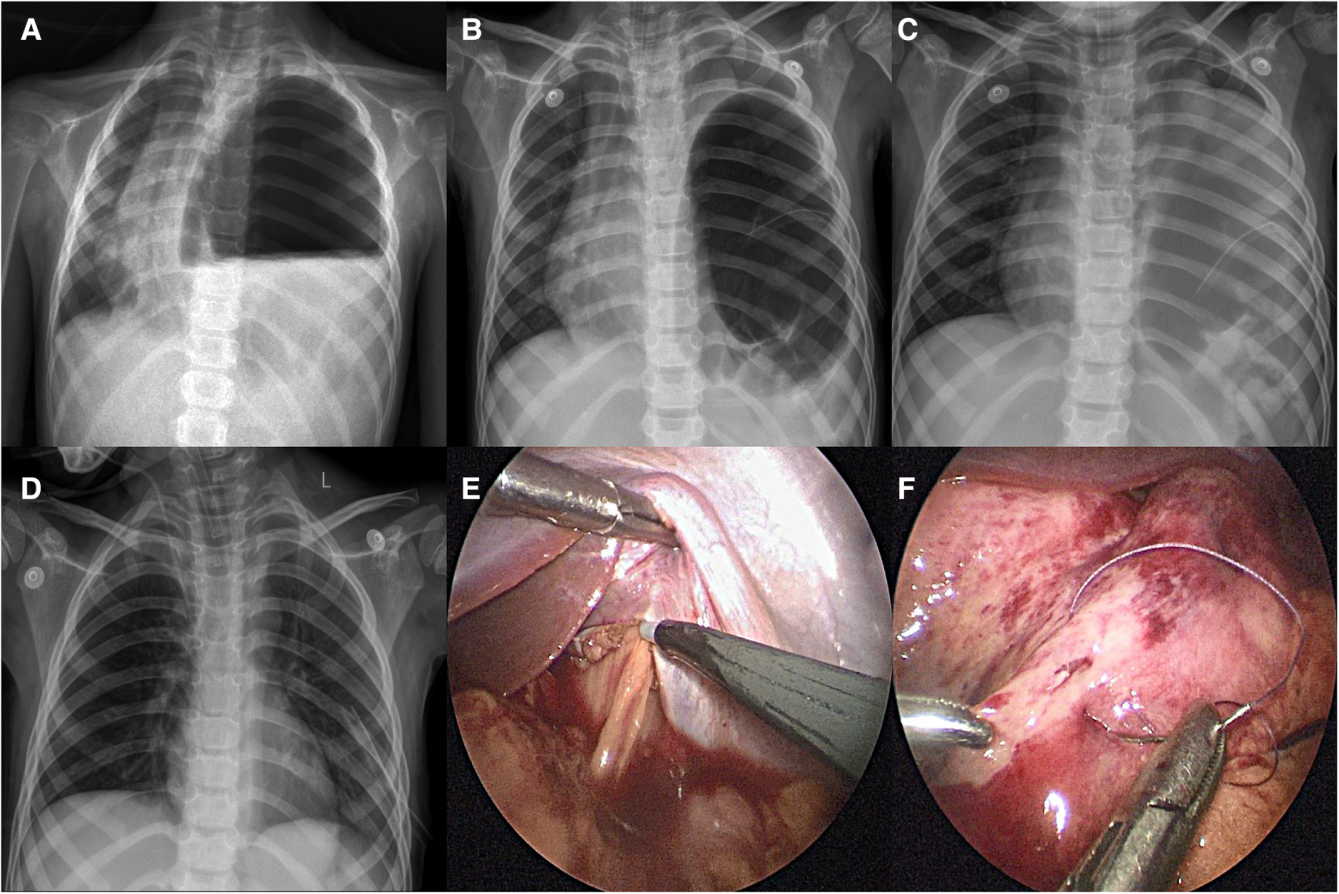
Case 1: preoperative and postoperative x-ray findings and intraoperative conditions: (A) encapsulated gas accumulation with mediastinal displacement; (B) encapsulated gas accumulation still exists after re-placing the thoracic drainage tube; (C) after adjusting the thoracic drainage tube, the wrapped gas accumulation disappeared, but the left costophrenic angle was vague, indicating the presence of pleural effusion; (D) after the operation, the diaphragm recovered to normal position and the lung expanded well; (E) hemorrhagic effusion in the abdominal cavity, obvious gastric edema and expansion, and enlarged diaphragm incision; (F) repair iatrogenic gastric perforation.
